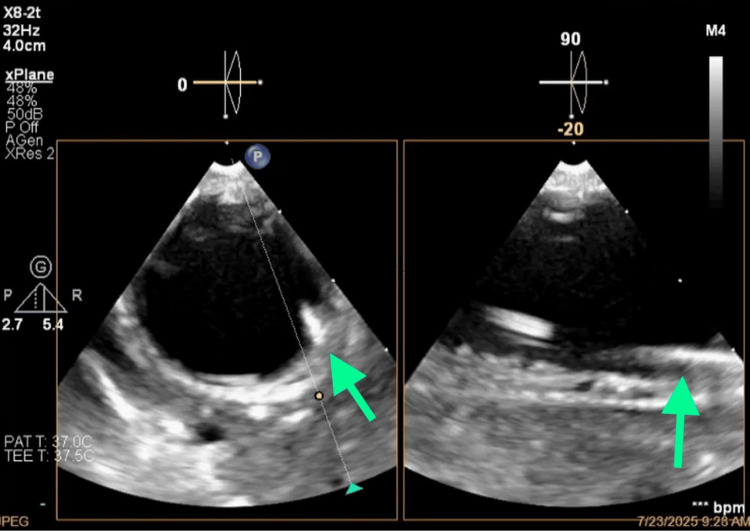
Ultrasound guidance of arterial cannulation.
